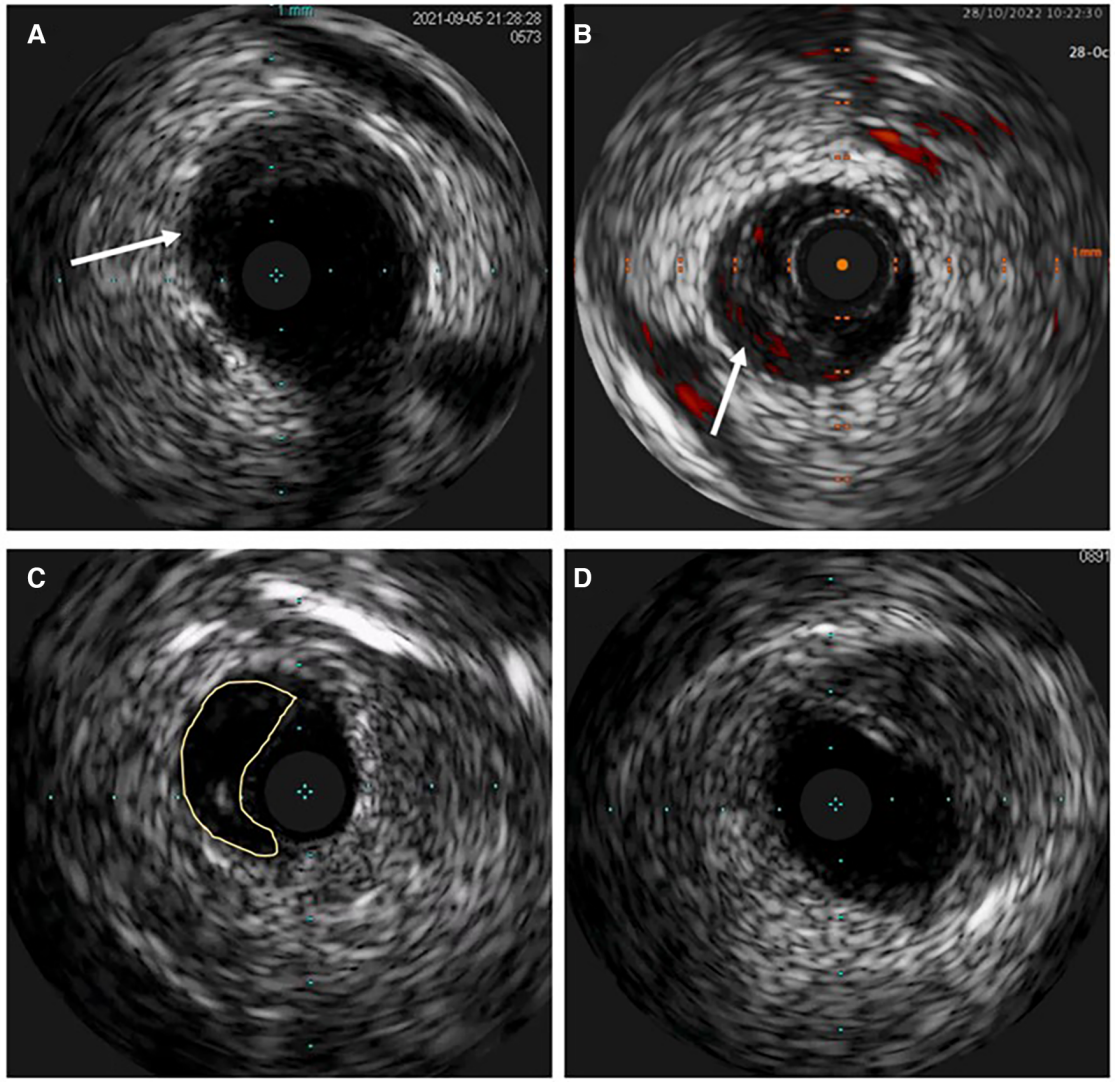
Intravascular ultrasound (IVUS) image of spontaneous coronary artery dissection (SCAD). (A) Intramural hematoma with the dissection entry (arrow). (B) ChromaFlow highlighting blood flow within intramural hematoma (IMH) with red color (arrow). (C) True and false lumen (yellow field) with the IVUS probe in the true lumen. (D) IMH in the left main resembles lipid-rich atheroma. Careful analysis of the entire pull-back length may be required in such cases.
